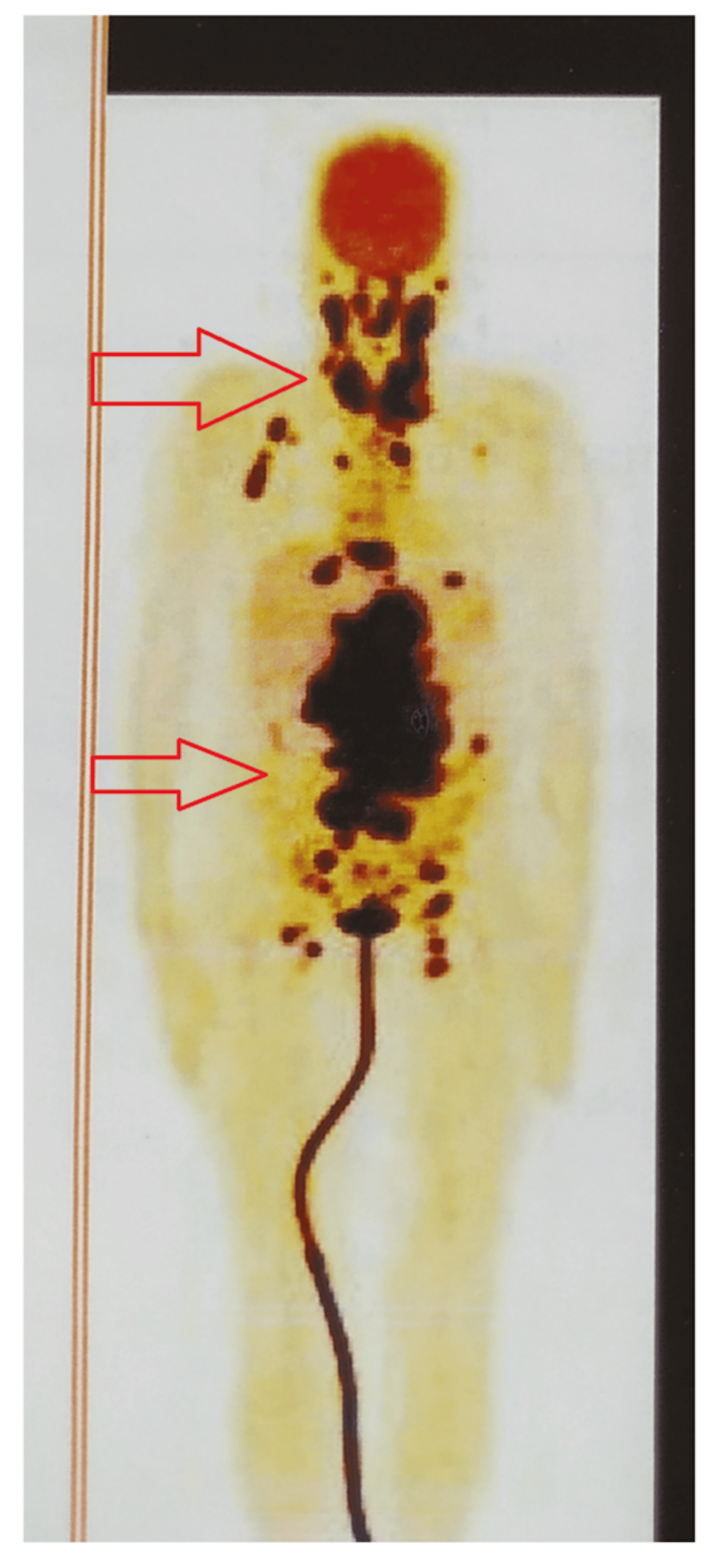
Pre-chemotherapy positron emission tomography-computed tomography scan showing supra and infra-diaphragmatic lymph nodes.
